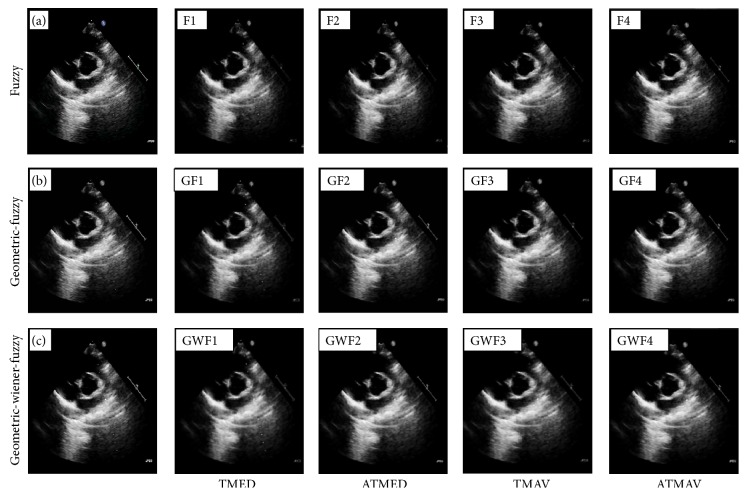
Denoised echocardiographic images: (a) original image, (b) geometric filter, (c) geometric-wiener filter, F1 to F4: TMED, ATMED, TMAV, and ATMAV, GF1 to GF4: geometric-fuzzy filters, and GWF1 to GWF4: geometric-wiener-fuzzy filters.
